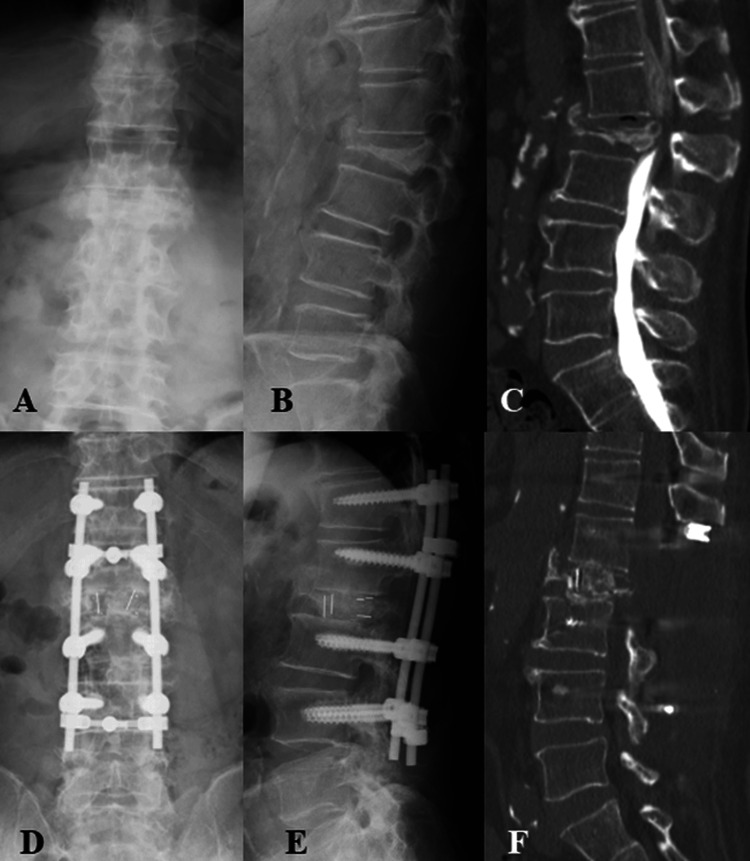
PAVREC Representative case of PAVREC surgery. A 72-year-old male patient who underwent posterior surgery for L2 osteoporotic vertebral collapse. posteroanterior (A) and lateral (B) radiographs and sagittal CT myelography (C). Postoperative posteroanterior (D) and lateral (E) radiographs, and CT sagittal image (F). PAVREC: posterior-approach vertebral replacement with rectangular parallelepiped cages, CT: computed tomography
